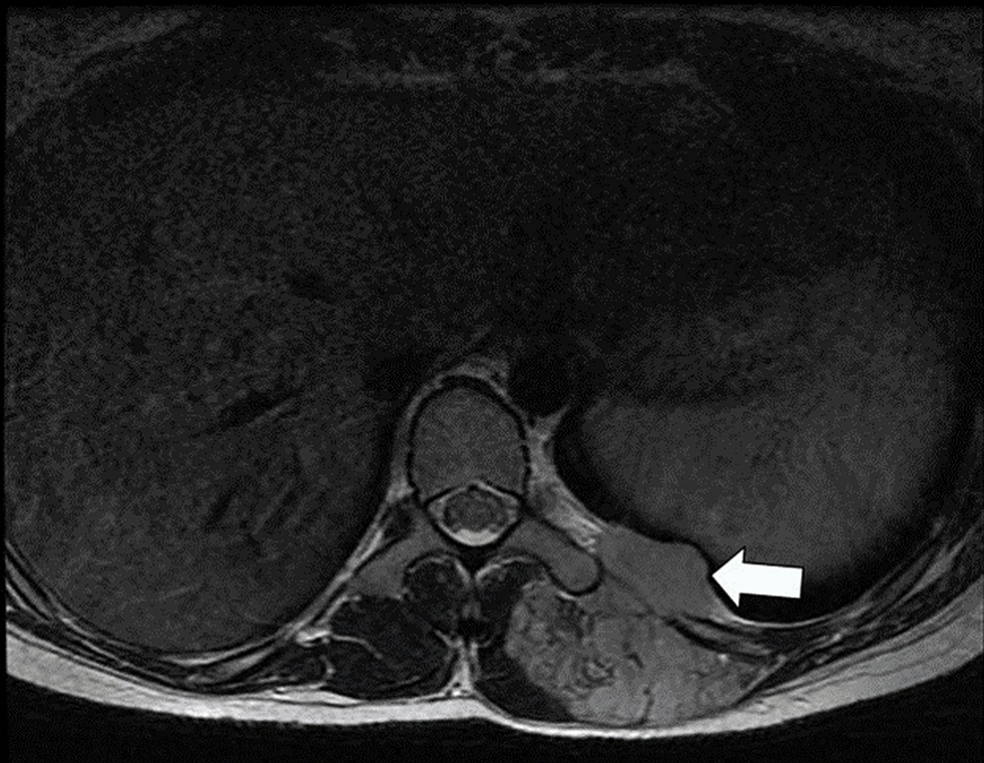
Axial T2-weighted MRI reveals a left paravertebral mass involving the left pleura (arrow).
